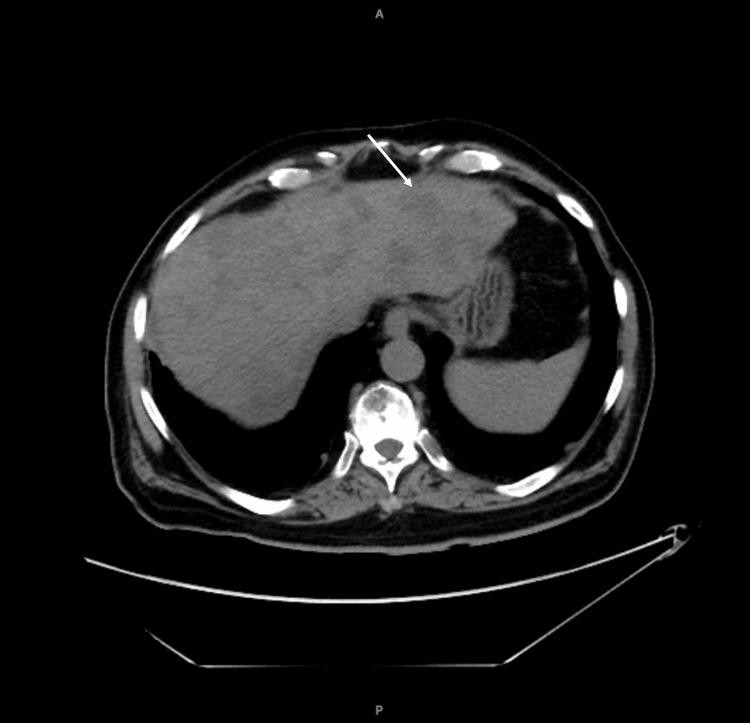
Computed tomography (CT) scans without contrast show numerous hepatic lesions
